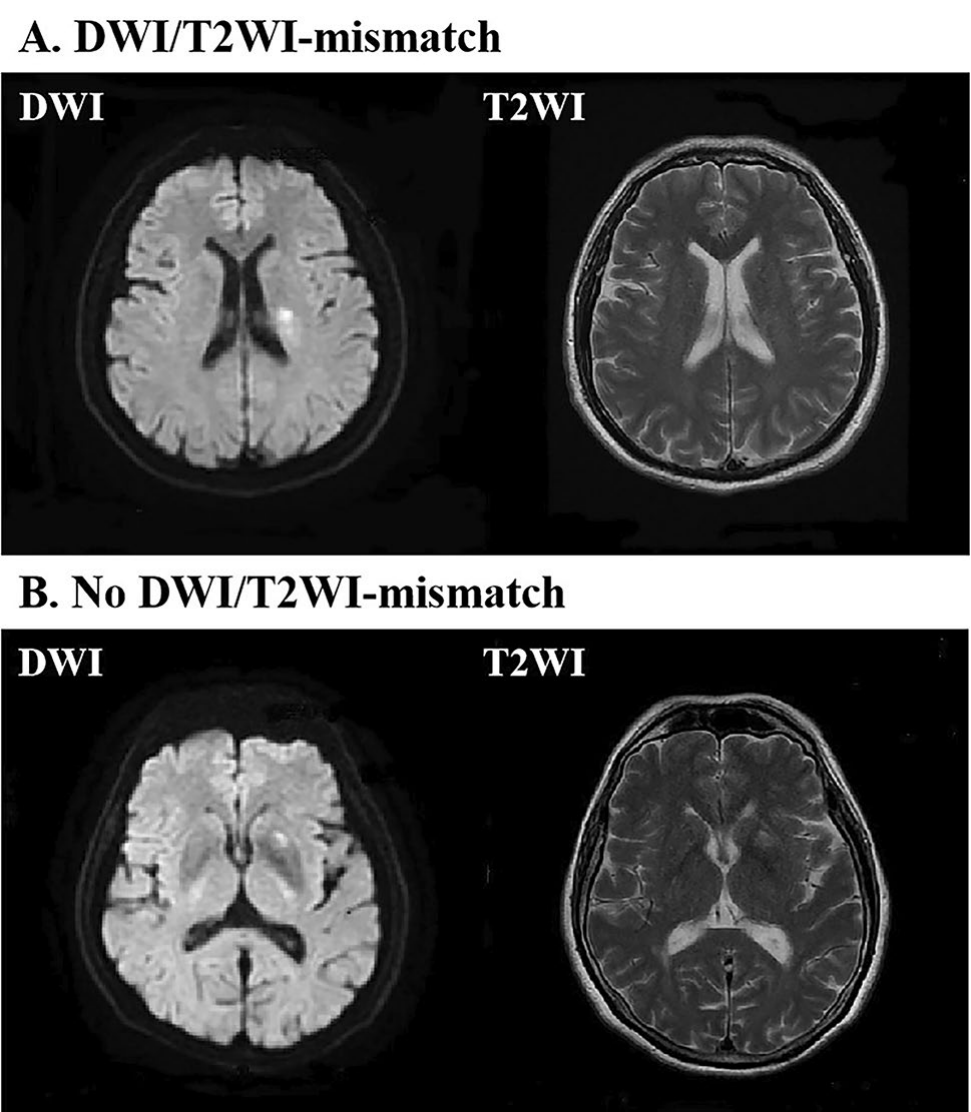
MRIs of patients with LSA stroke, with or without mismatch between DWI and T2WI within 12 h after onset of symptoms. (A) Pairs of images showing acute ischemic lesions on DWI but not on T2WI imaging (i.e., with DWI/T2WI-mismatch). (B) Pairs of images showing acute ischemic lesions on DWI together with a corresponding hyperintensity on T2WI imaging (without DWI/T2WI-mismatch).
